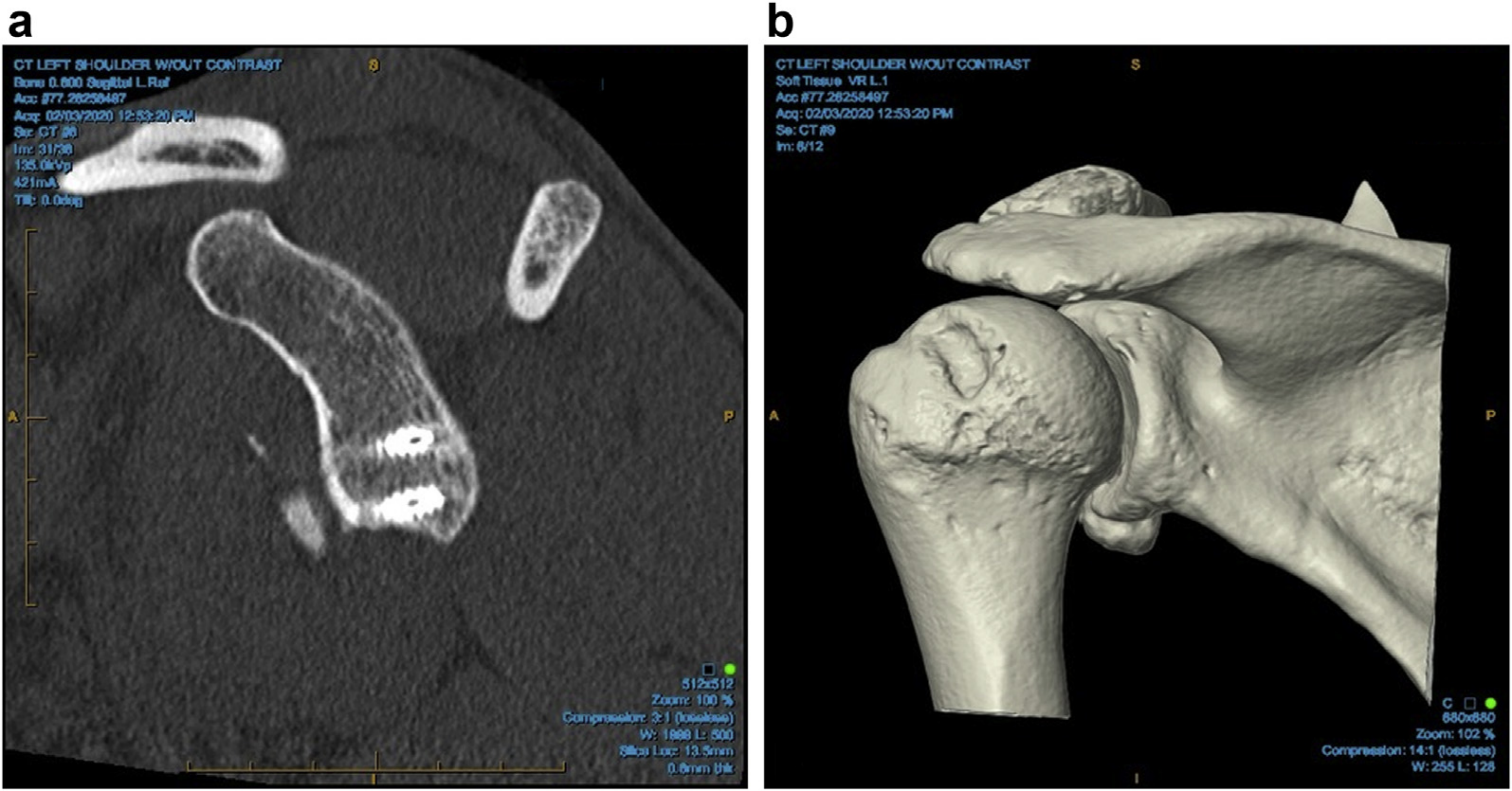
Unicortical screw fixation on (a) CT and (b) 4D CT scan. *CT*, computed tomography.
